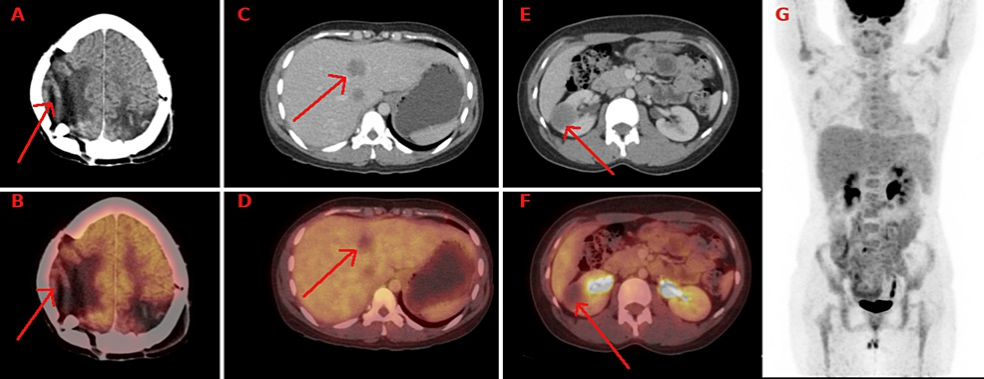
Cross-sectional CT image (A) and fused PET CT image (B) reveal postoperative changes in the right high frontoparietal lobe with no evidence of abnormal FDG avid lesion. Cross-sectional CT image (C) and fused PET CT image (D) reveal non-FDG avid hypodense lesions in the liver, suggesting healed lesions. Cross-sectional CT image (E) and fused PET CT image (F) reveal a non-FDG avid hypodense lesion involving the right kidney. Previously noted lesion involving jejunal bowel loops is not seen in the post-therapy images. Maximum intensity projection (MIP) image G reveals the resolution of previously noted foci of increased FDG uptake involving the liver, bilateral kidneys, bowel loops, and lung regions, suggesting a response to chemotherapy.
